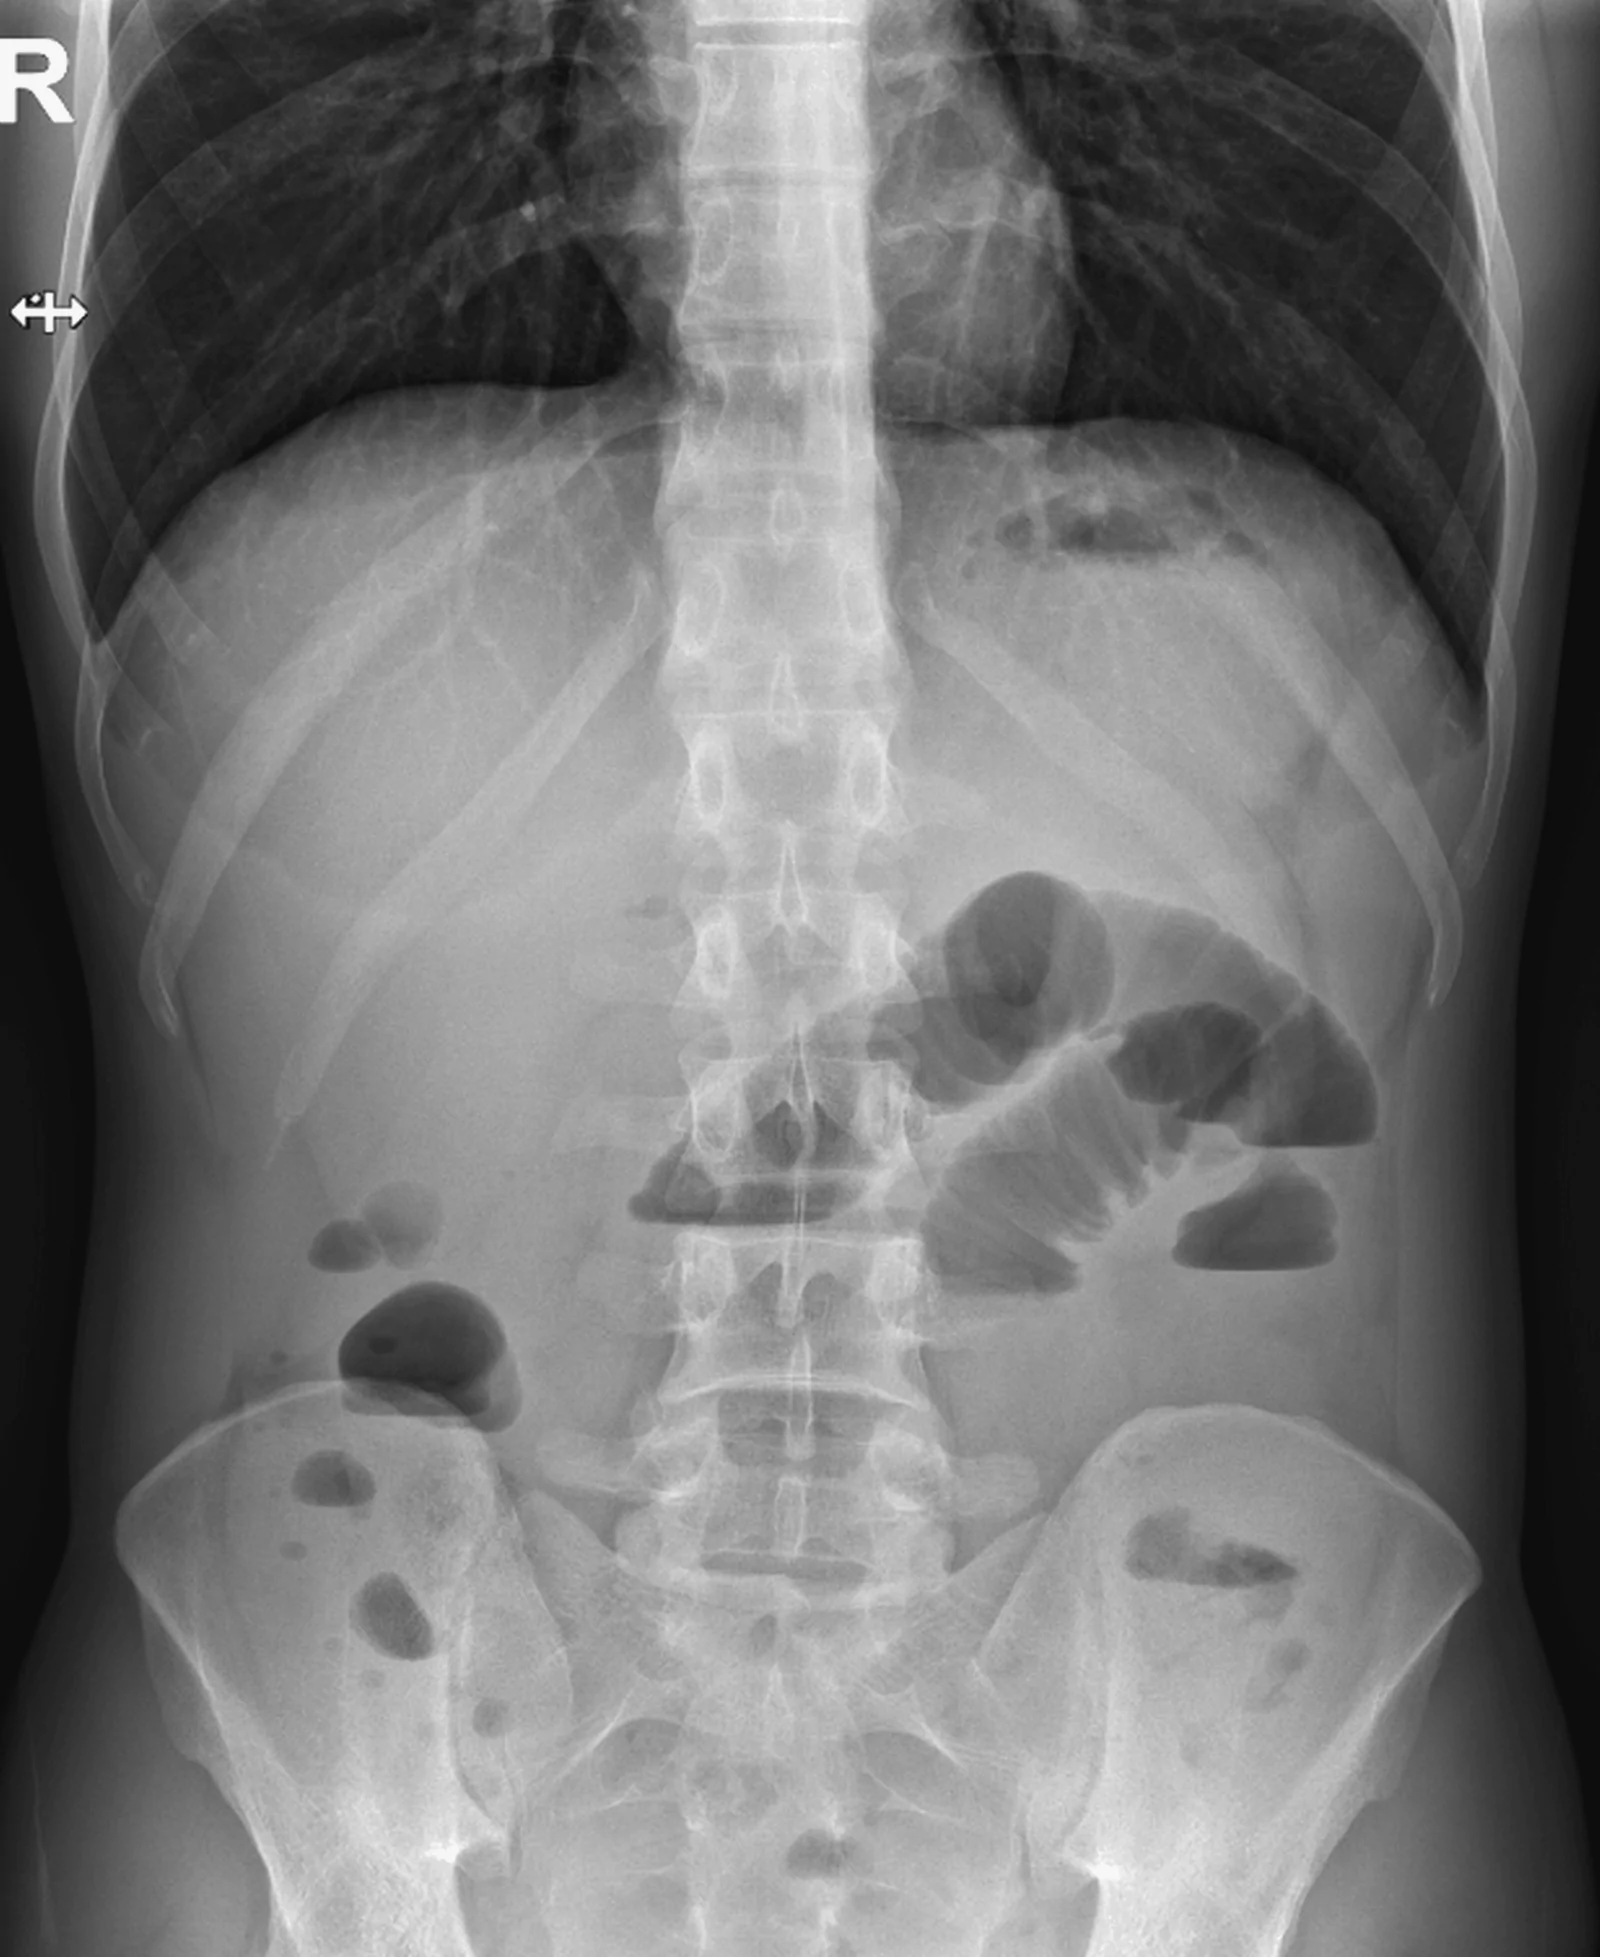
Upright plain abdominal radiograph showing multiple air-fluid levels and dilated small bowel loops measuring up to approximately 40 mm, consistent with mechanical small bowel obstruction
